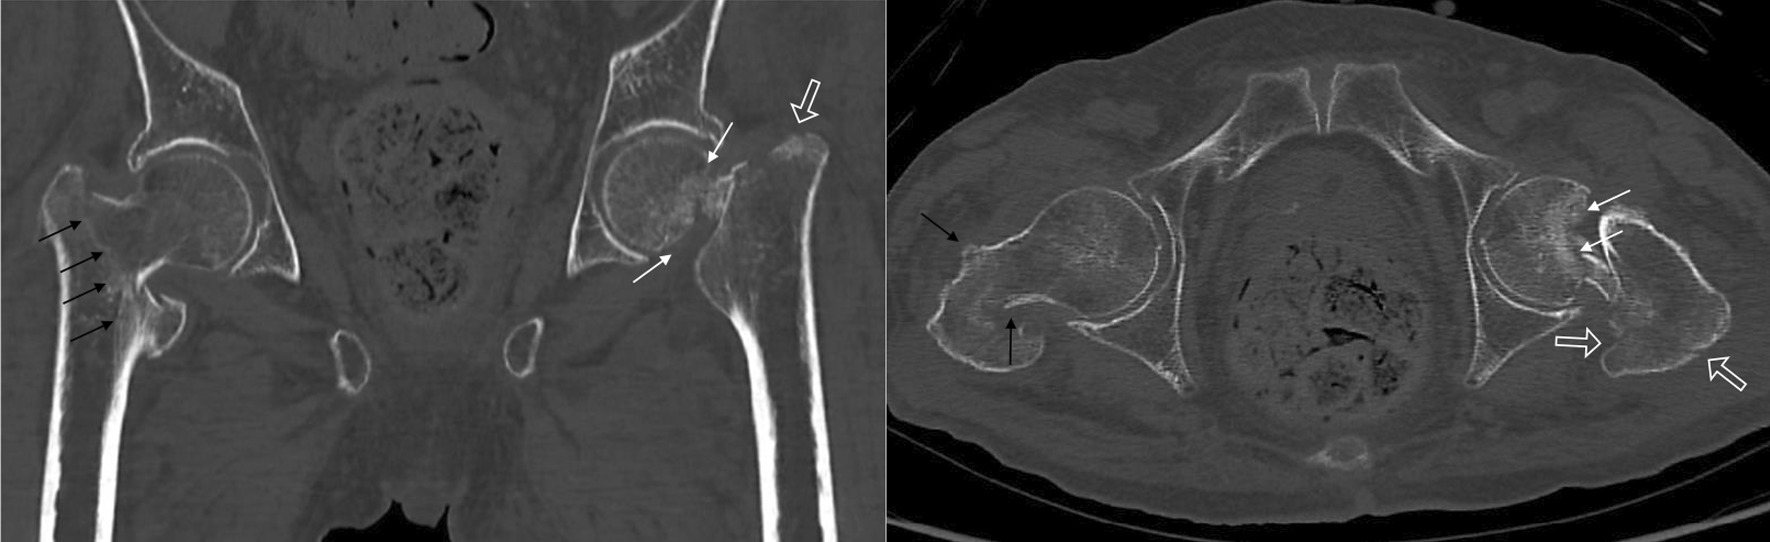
Computed tomography (CT) results showing bone osteosclerosis at the margin of the femoral head (white arrow), indicating that the left femoral neck fracture was an old fracture, while the left greater trochanteric fracture (white outline arrow) and right trochanteric fracture (black arrow) were fresh fractures
